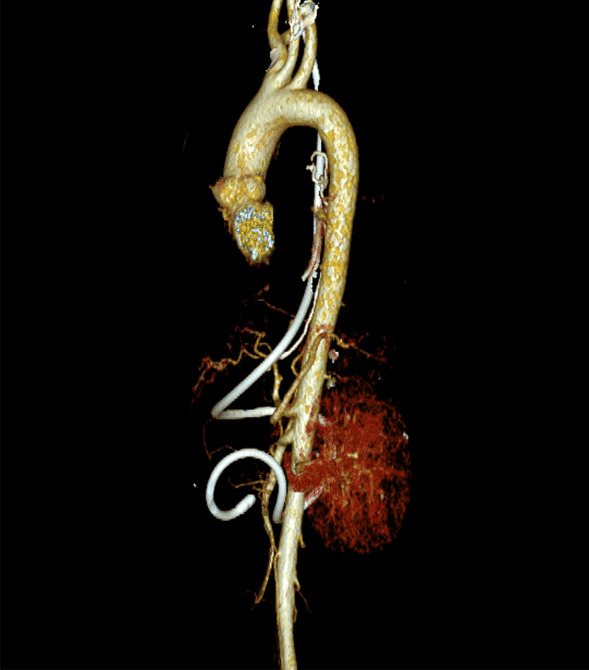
CTA of the whole aorta on the 19th day
